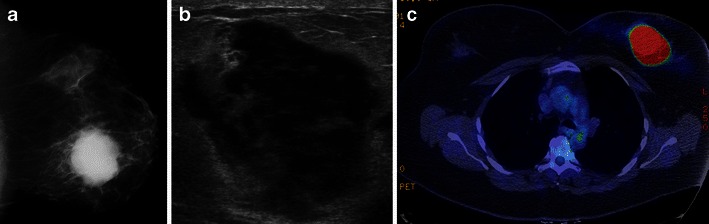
A 45-year-old woman with primary B-cell lymphoma presents with a palpable mass in the left breast. a Left cranio-caudal mammogram demonstrates a high-density irregular mass with indistinct margins in the medial left breast. b Ultrasound of the palpable mass demonstrates an irregular hypoechoic mass with thin echogenic rim. c Axial PET/CT shows homogeneous intense hypermetabolic activity within the mass. No uptake is seen within normal appearing nodes in the left axilla (*not shown*)
